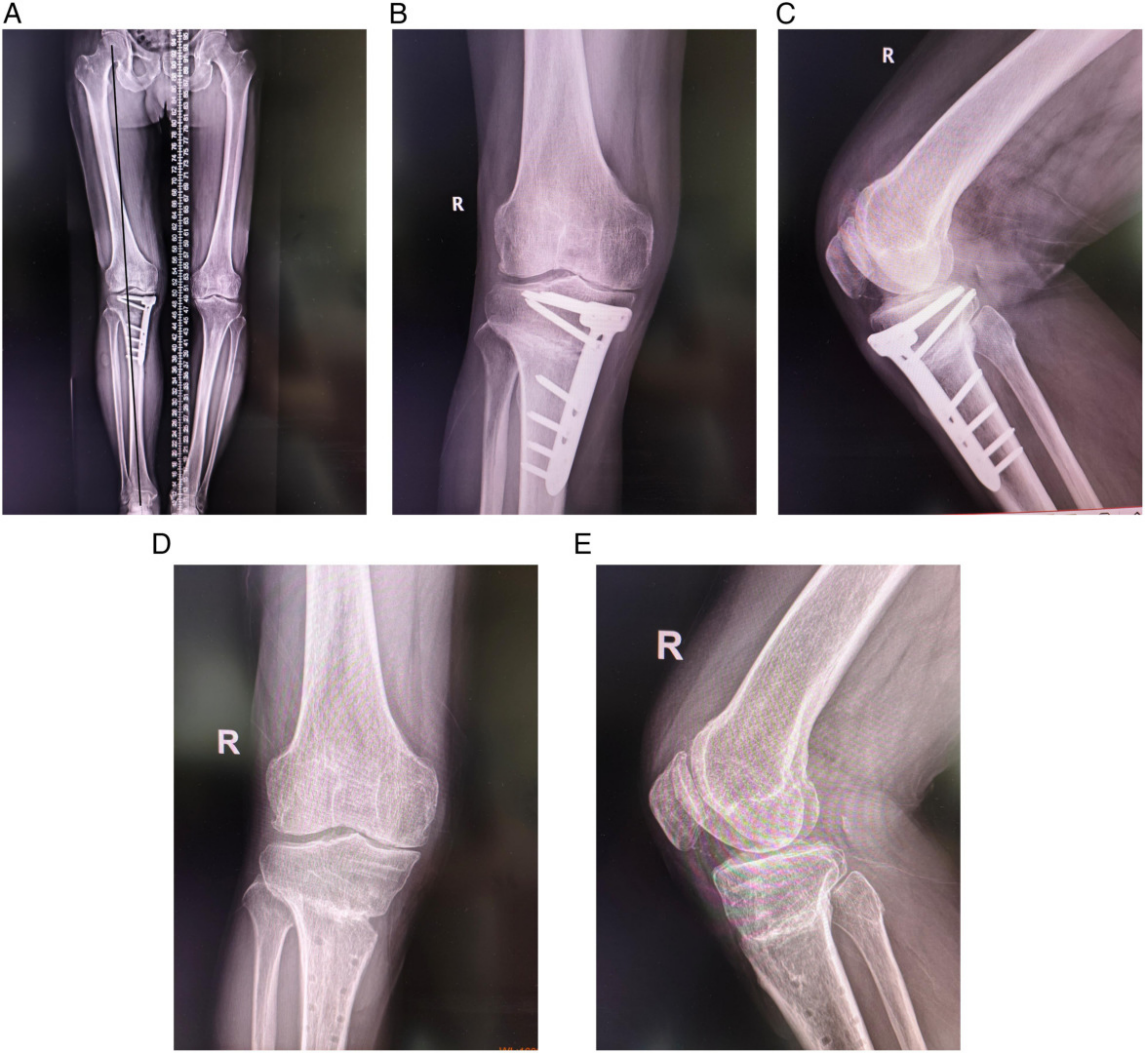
The imaging data were reviewed at each stage after operation. (A) Full-length radiographs of both lower limbs 5 days after surgery showed good orthopedic results; (B,C) 6 months postoperatively, X-ray films showed that the osteotomy site healed well; (D,E) the plate for internal fixation was removed 1 year after surgery.
